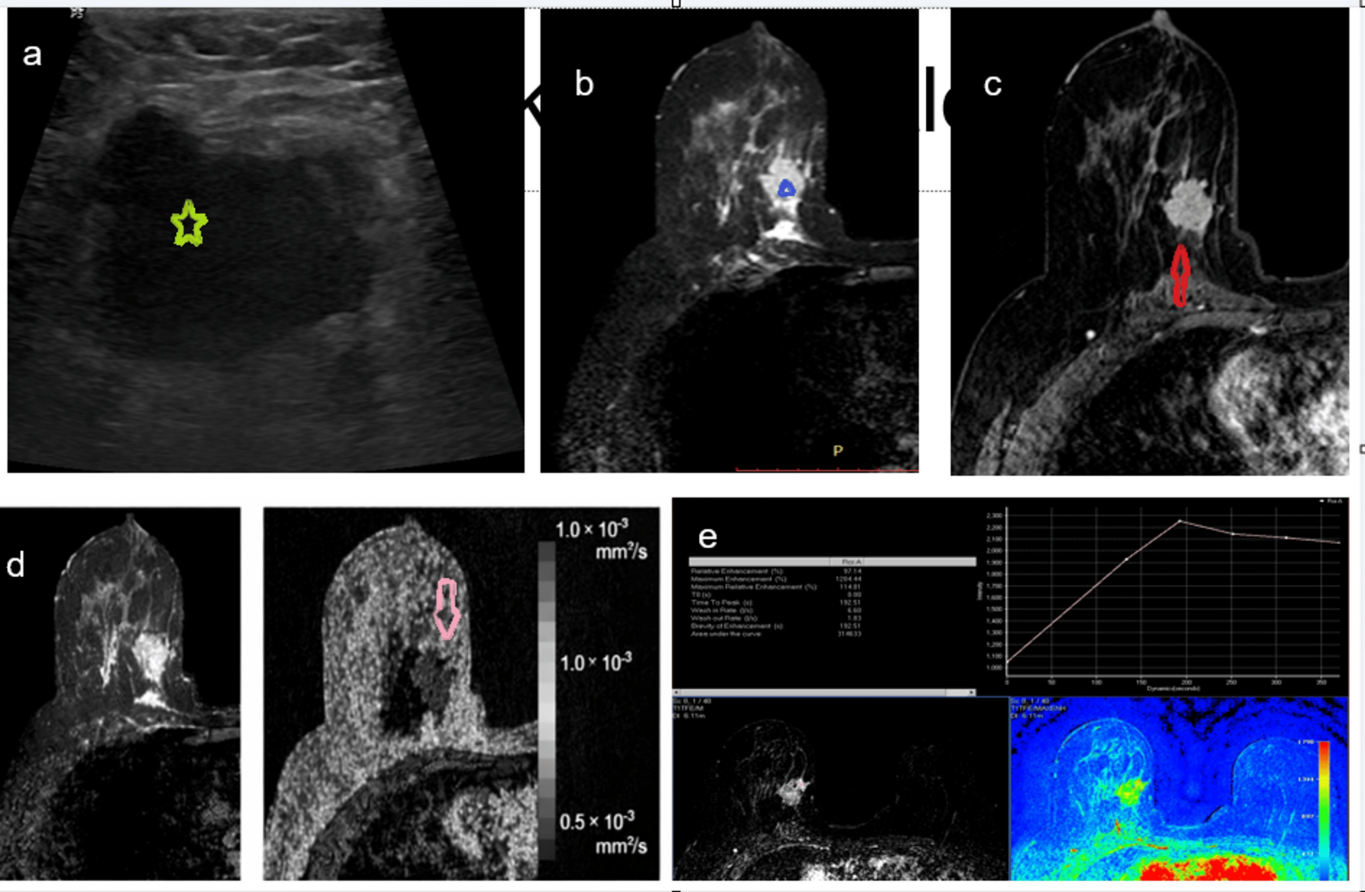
Multimodality imaging evaluation of a breast lesion (a) Ultrasound shows an irregular hypoechoic solid mass with irregular margins and no posterior acoustic features (green star).
